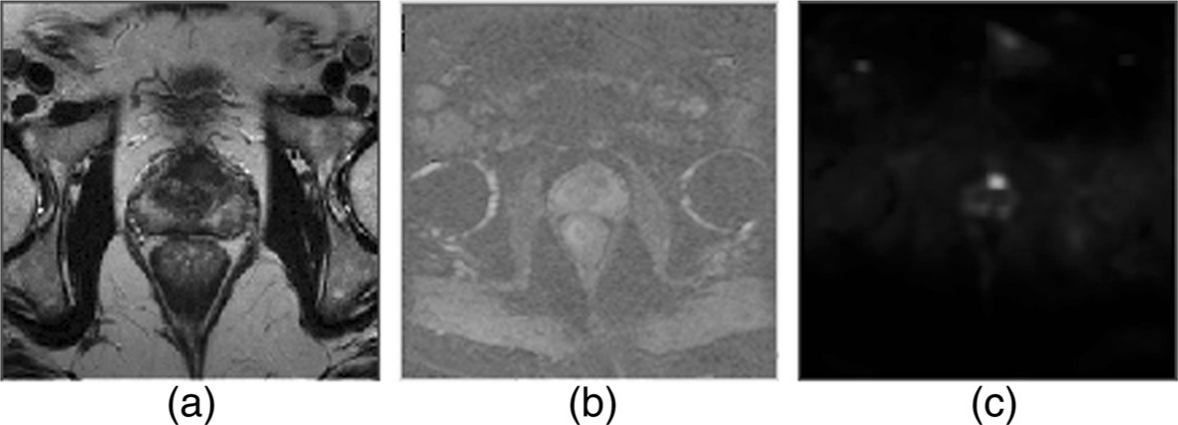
Example case 2. Tumor stands out well on CDI and not at all on T2-weighted imaging in patient with prostate cancer. a), T2-weighted imaging shows no change in signal towards left side of transition zone. b), ADC map shows increased contrast around left side of transition zone. c), CDI shows very high signal intensity corresponding to left side of transition zone.
